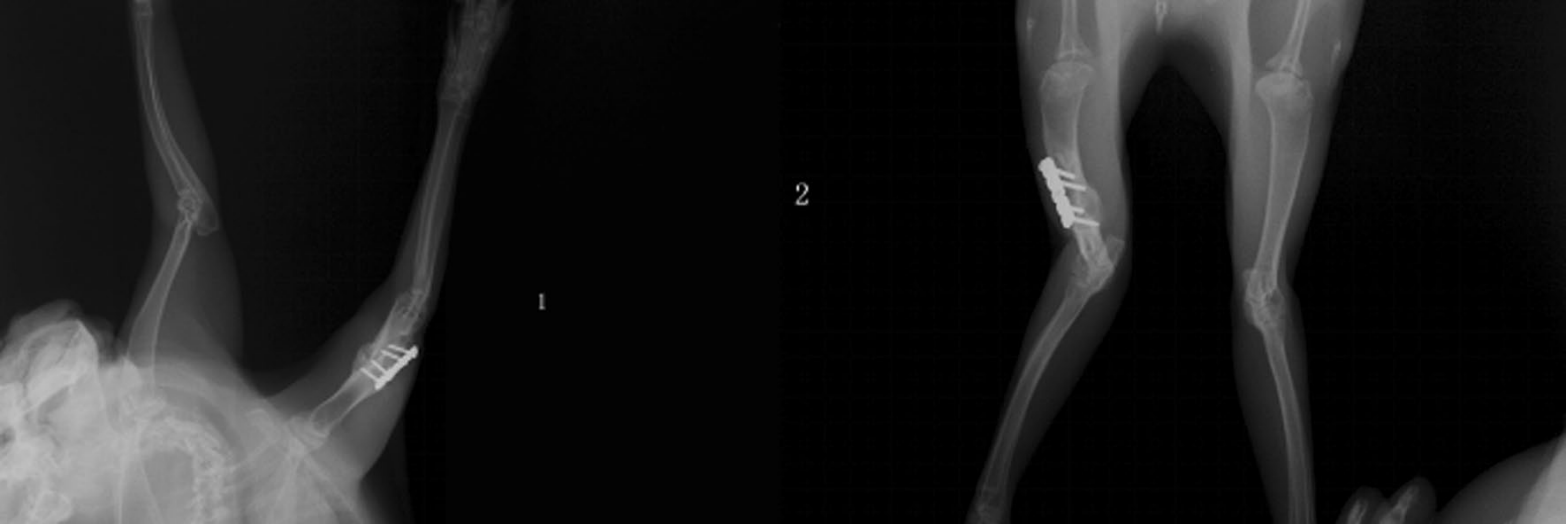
Postoperative X-ray analysis to confirm bone healing.
